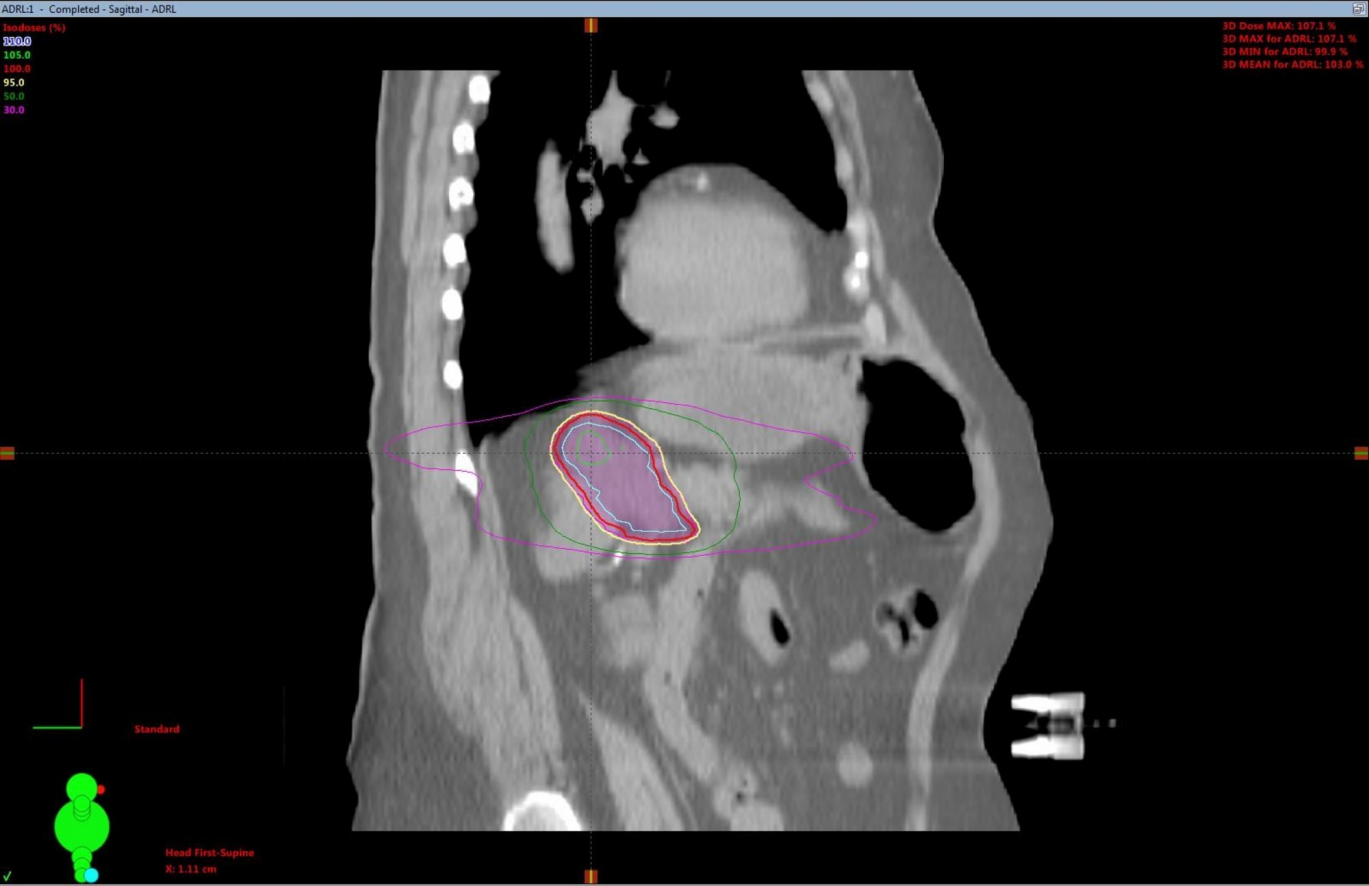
Adrenal oligometastasis stereotactic body radiotherapy (SBRT) planning computed tomography (CT) sagittal view.
